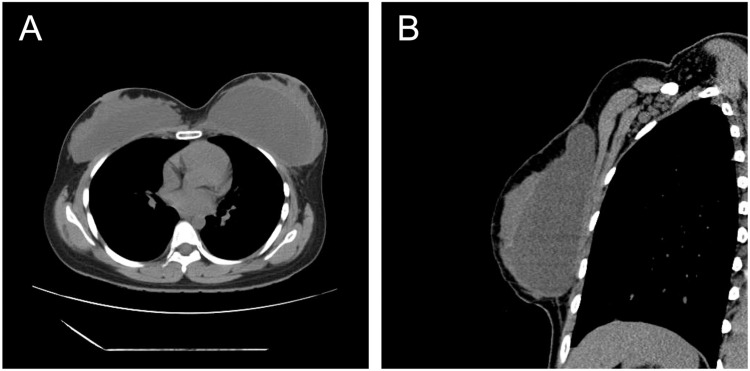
Computed Tomography (CT) image obtained prior to surgical removal. (A): Axial CT image. The left breast with the copolyamide filler appears swollen. (B): Sagittal CT image. The copolyamide filler is localized between the posterior mammary gland and the anterior pectoralis major muscle.
